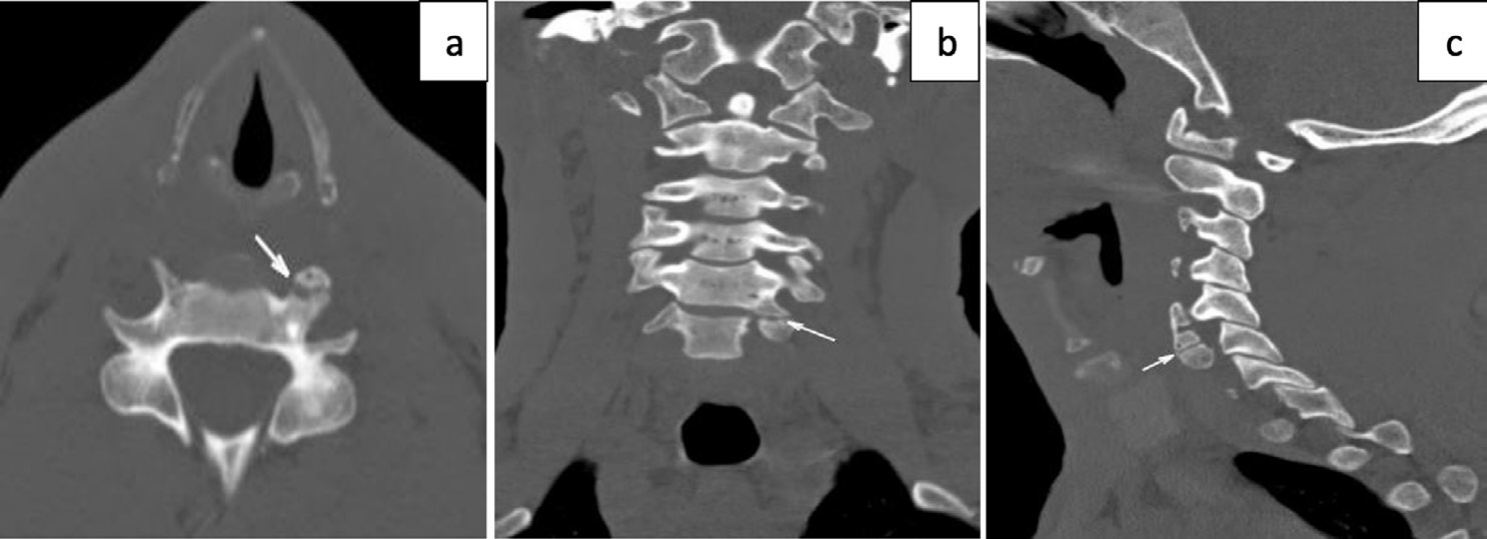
34-year-old male following a mob-assault. Cervical CT with axial, coronal and sagittal reformations shows an incidental left-sided elongation of the anterior tubercle of the C5/C6 transverse process with an accessory articulation.
